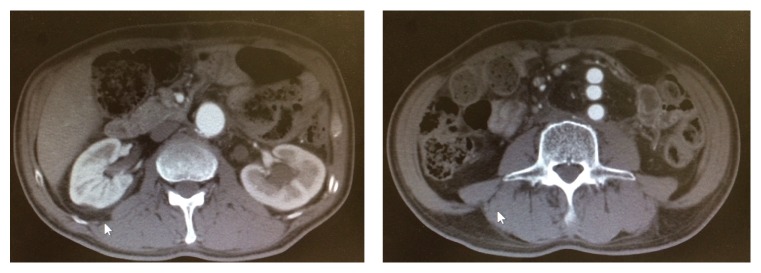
Computed tomography scans taken when the patient was discharged. No leakage of blood into the area around the artificial vascular graft or residual abscess was seen. The omentum with intact blood flow can be seen in the area around the graft.
